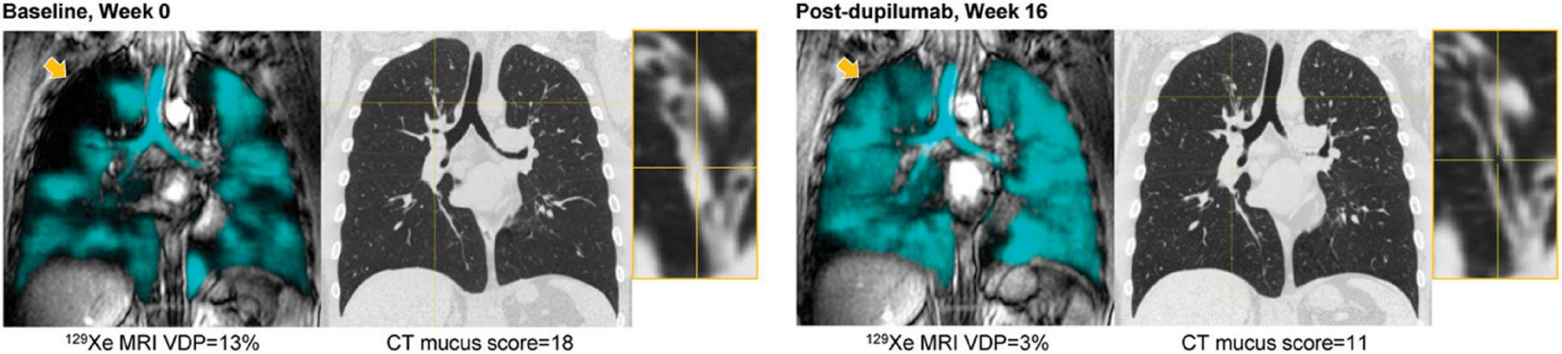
^129^Xe ventilation MRI (cyan) and CT coronal slices at baseline and the end of dupilumab treatment (week 16) for a 40-year-old man. CT mucus score and MRI ventilation were improved but not normalized by dupilumab. Yellow crosshairs identify a mucus plug in the apical segment of the right upper lobe and a distal ventilation defect (arrow) at baseline that were resolved after dupilumab administration. Adapted with permission from Svenningsen S et al. *Am J Respir Crit Care Med* 2023;208:995–997 [30] CT, computed tomography; MRI, magnetic resonance imaging; VDP, ventilation defect percentage
